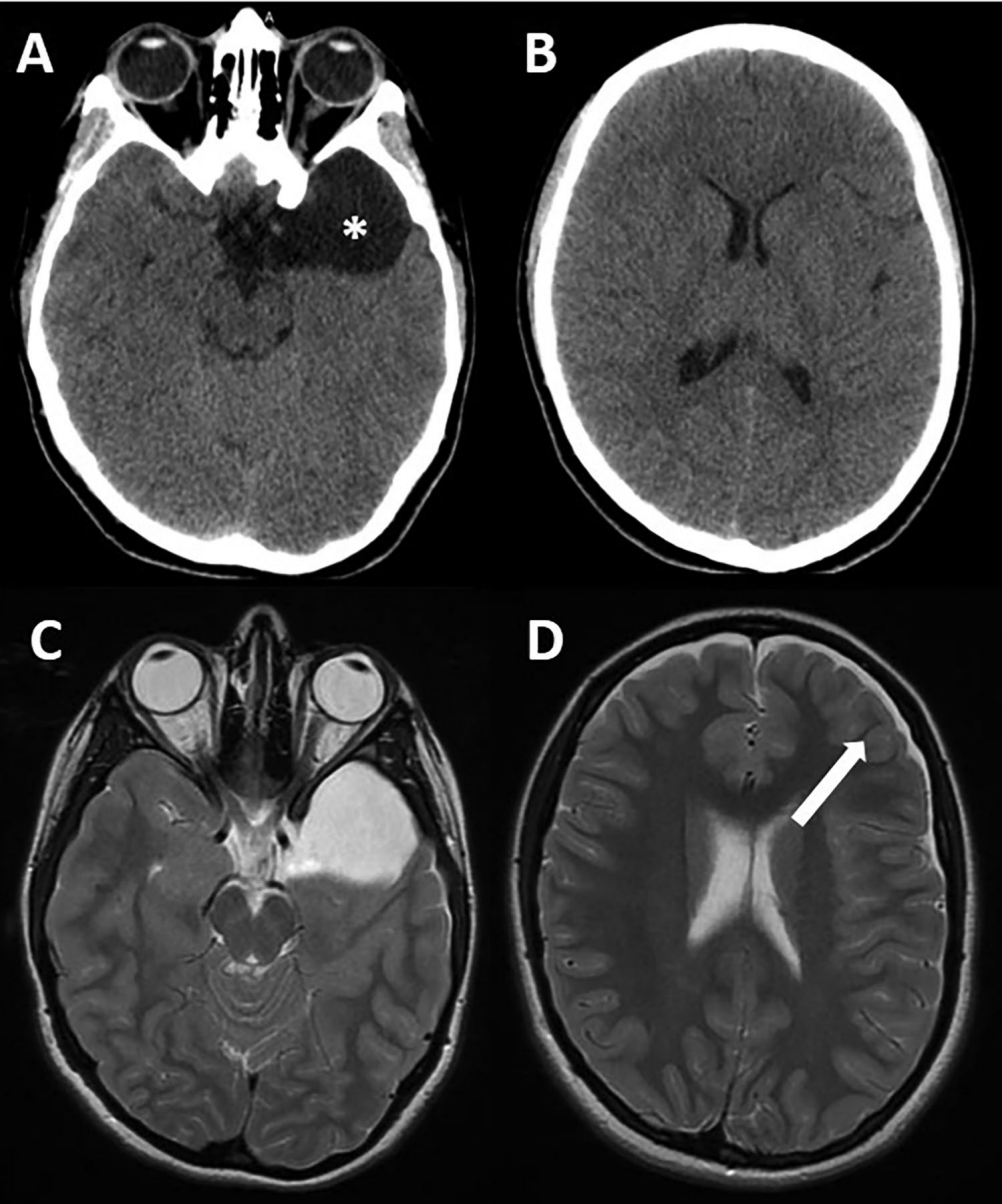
A, B CT scan showing the left SAC (asterisk); C, D MRI (axial T2 view) showing the persistence of the cyst and the appearance of a hygroma (arrow)
